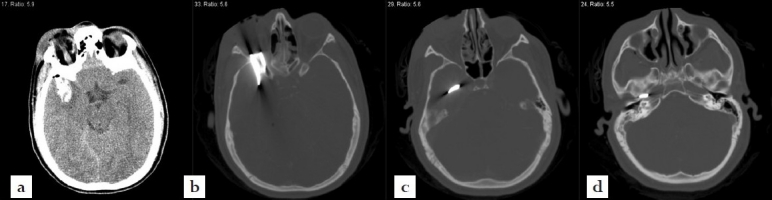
Axial noncontrast CT scans of the head (a) soft tissue window and (bd) bone window) demonstrating the retained metal fragment measuring ca. 5 cm × 1.5 cm × 0.2 cm.
